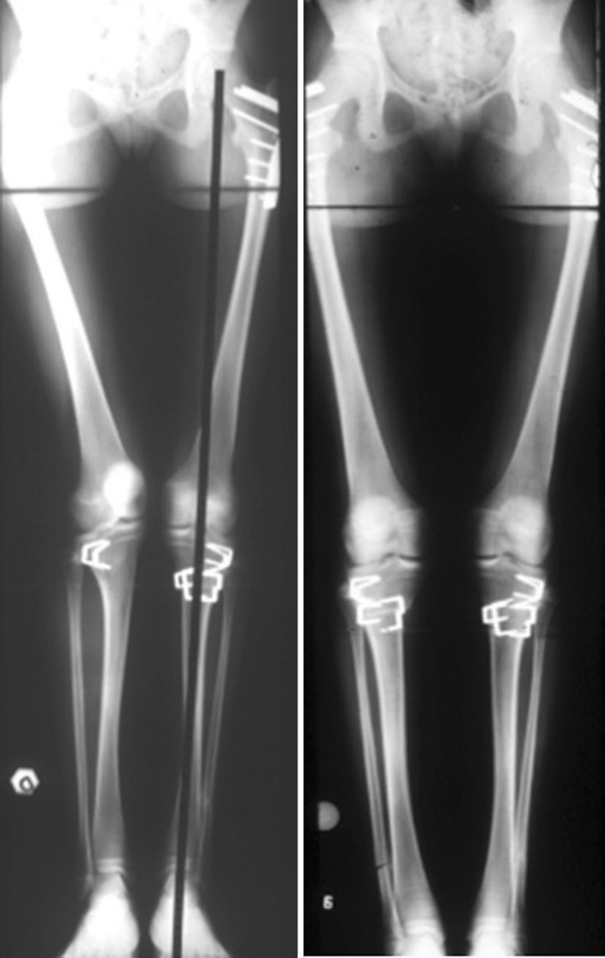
Patient GS. Radiograph after correction: proximal osteotomy of the femur fixed with blade-plate and screws and proximal tibial osteotomy fixed with staples
